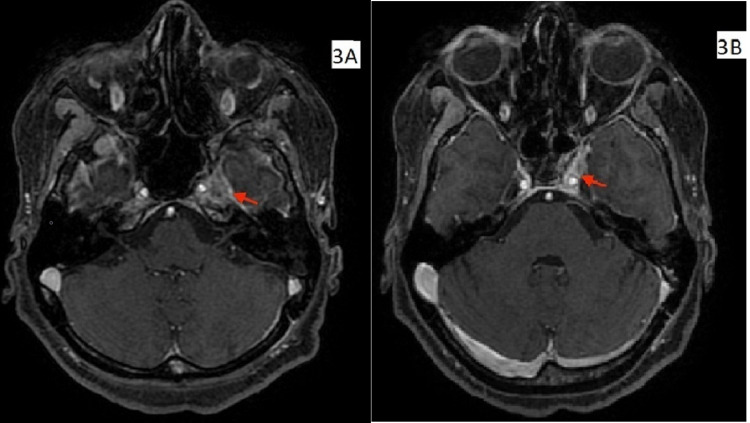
3A, 3B. Post-contrast T1-weighted axial images of contrast-enhanced magnetic resonance imaging of brain showing enhancing lesion along lateral wall of left cavernous sinus and left petrous apex, also involving left foramen ovale and left mandibular nerve with diffuse enhancing dural thickening and multifocal enhancing plaque-like dural thickening up to 4 mm along left cerebral convexity in insular region (red arrows).
